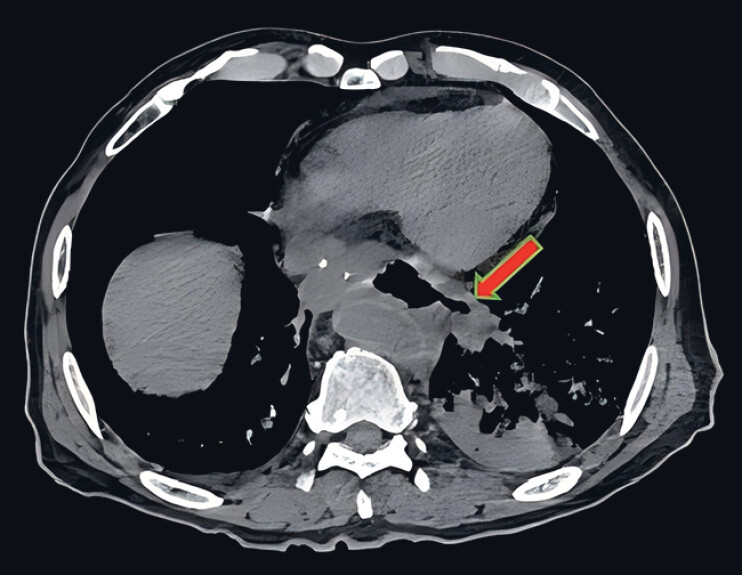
Preoperative chest computed tomography showed a thoracogastric tract connecting the left lower lobe bronchus through the mediastinum.
